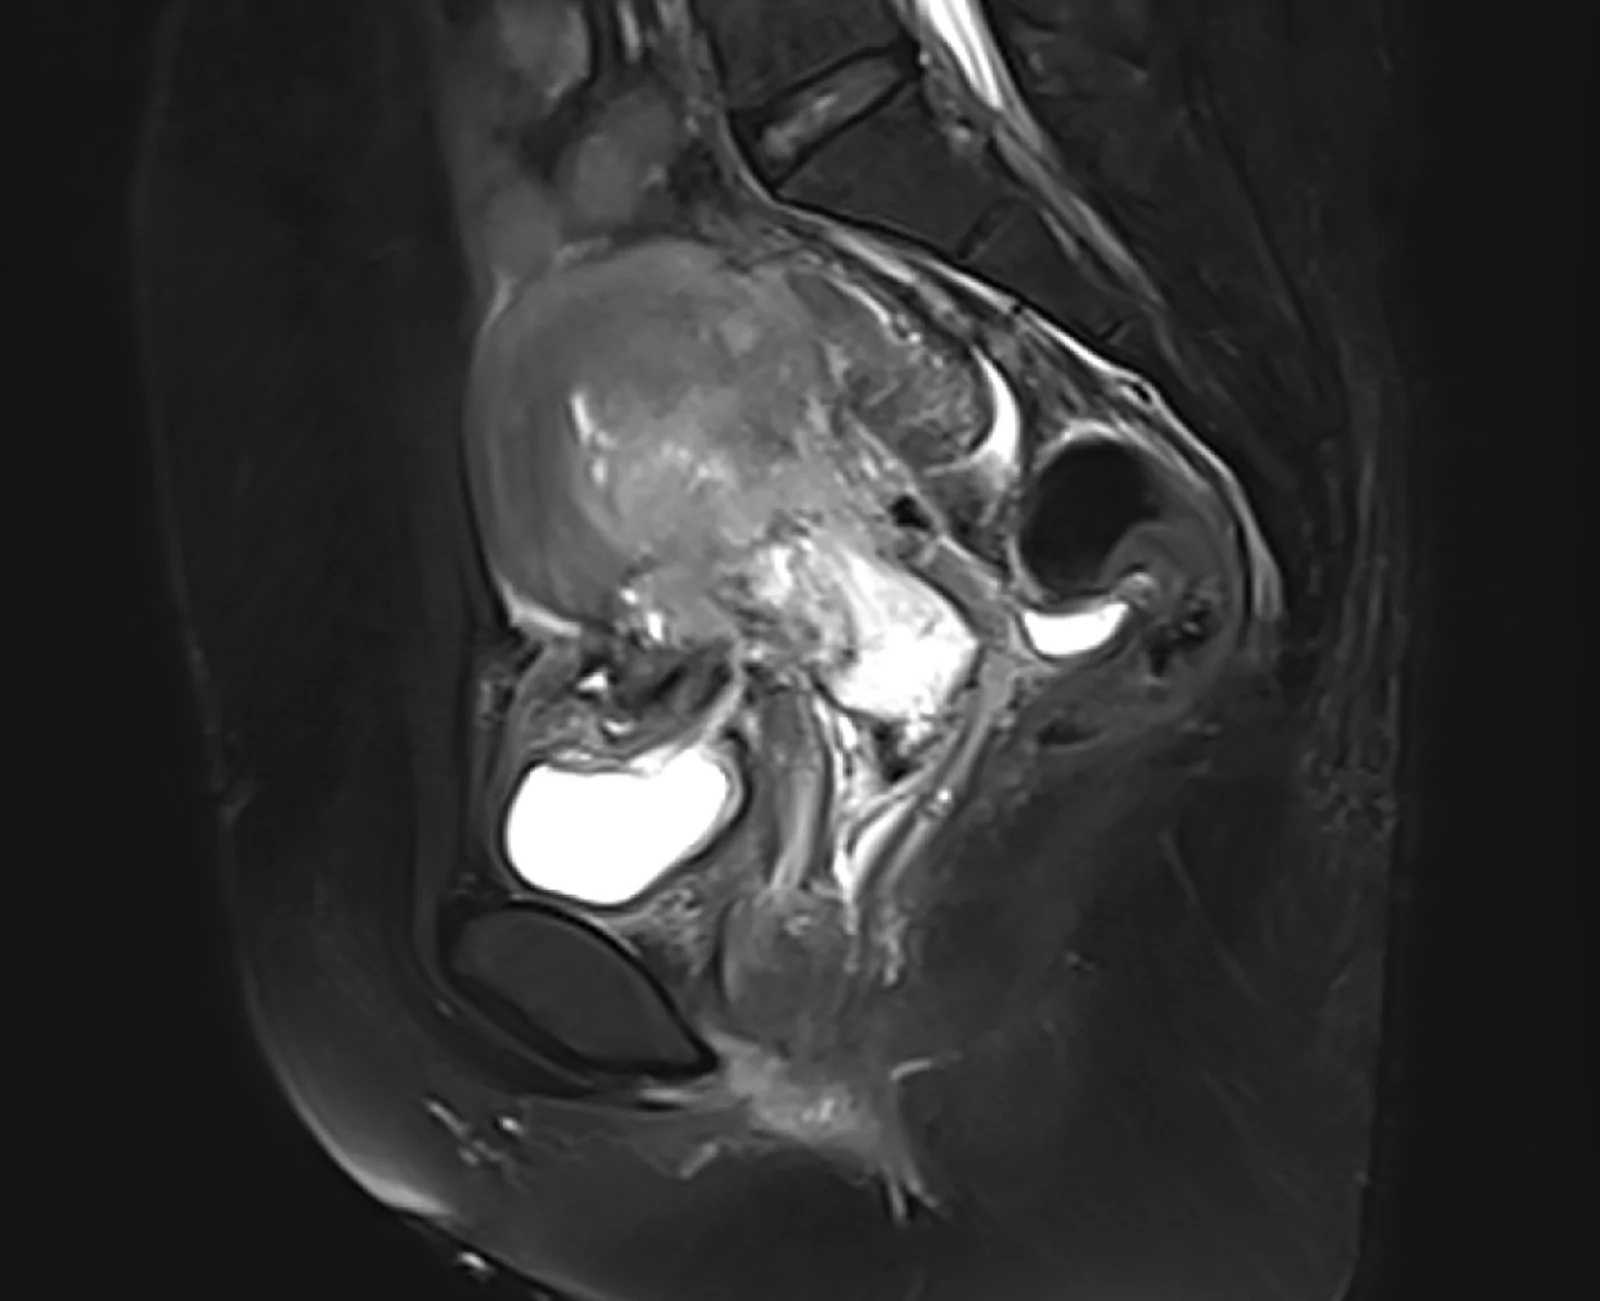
Post-NACT pelvic MRI. Sagittal T2-weighted fat-suppressed sequence obtained after 3 cycles of NACT (TP regimen) shows marked tumor shrinkage to 7.1 × 6.5 × 6.4 cm (arrows), corresponding to PR. The residual lesion remains hyperintense with irregular margins. MRI = magnetic resonance imaging, NACT = neoadjuvant chemotherapy, PR = partial remission, TP = paaclitaxel plus cisplatin.
